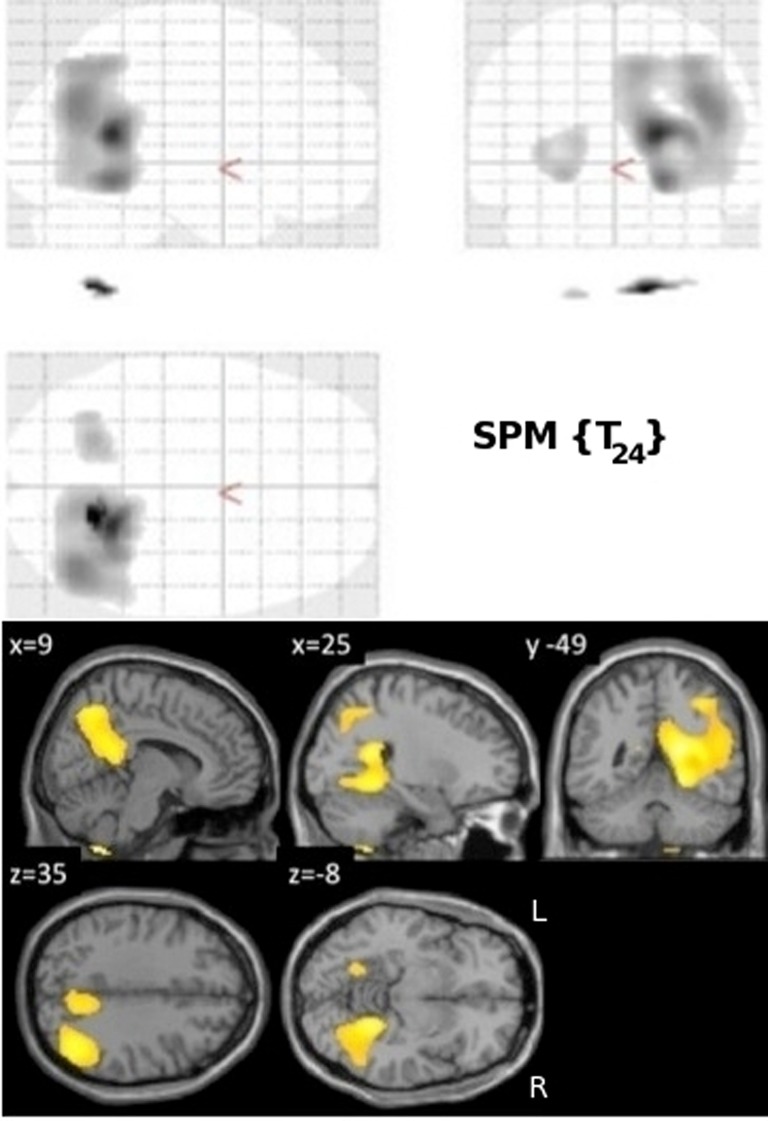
Results of the univariate linear regression of VRLT error score with FDG metabolism.
